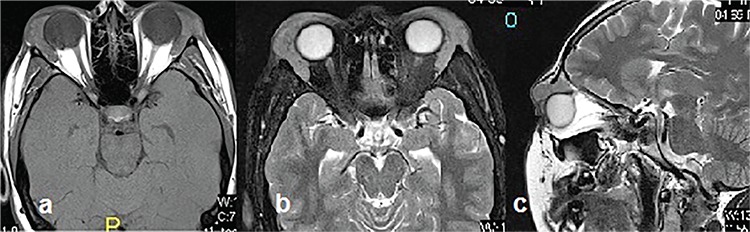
Magnetic resonance imaging showed bilateral symmetrical diffuse enlargement of the lacrimal glands and involvement of the superior eyelids and anterior orbit: isointense signal intensity relative to muscle on T1-weighted axial image (a); hypointense signal intensity on T2-weighted axial image (b); hypointense signal intensity on T2-weighted sagittal image (c)
